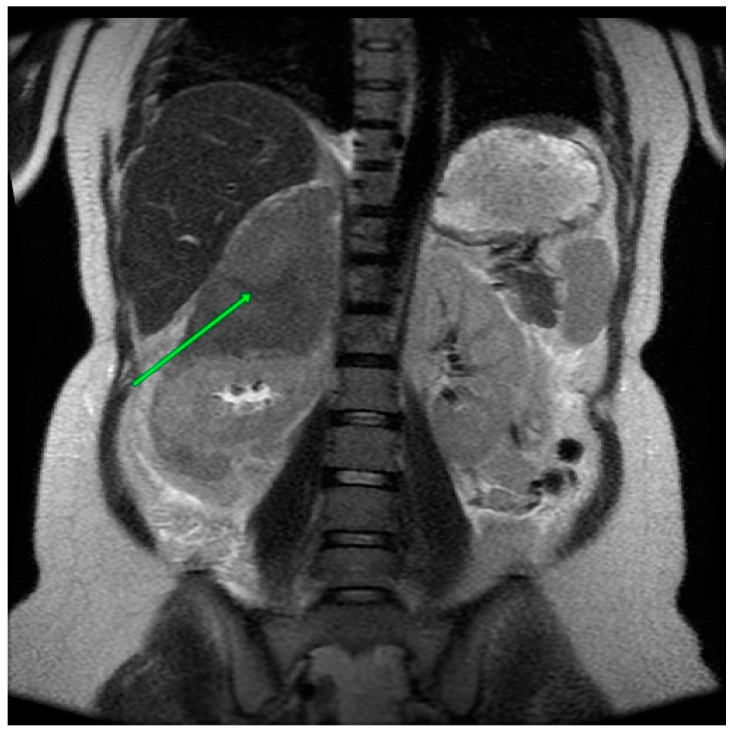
An abdominal MRI, revealed a hematoma in the right adrenal gland.
